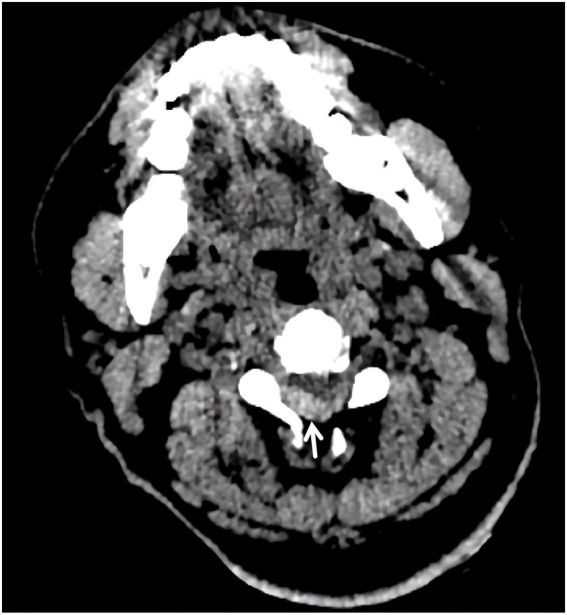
Cranial computed tomography angiography. An epidural lesion is visible within the cervical canal (white arrow). This finding was initially missed during hyperacute stroke assessment.
